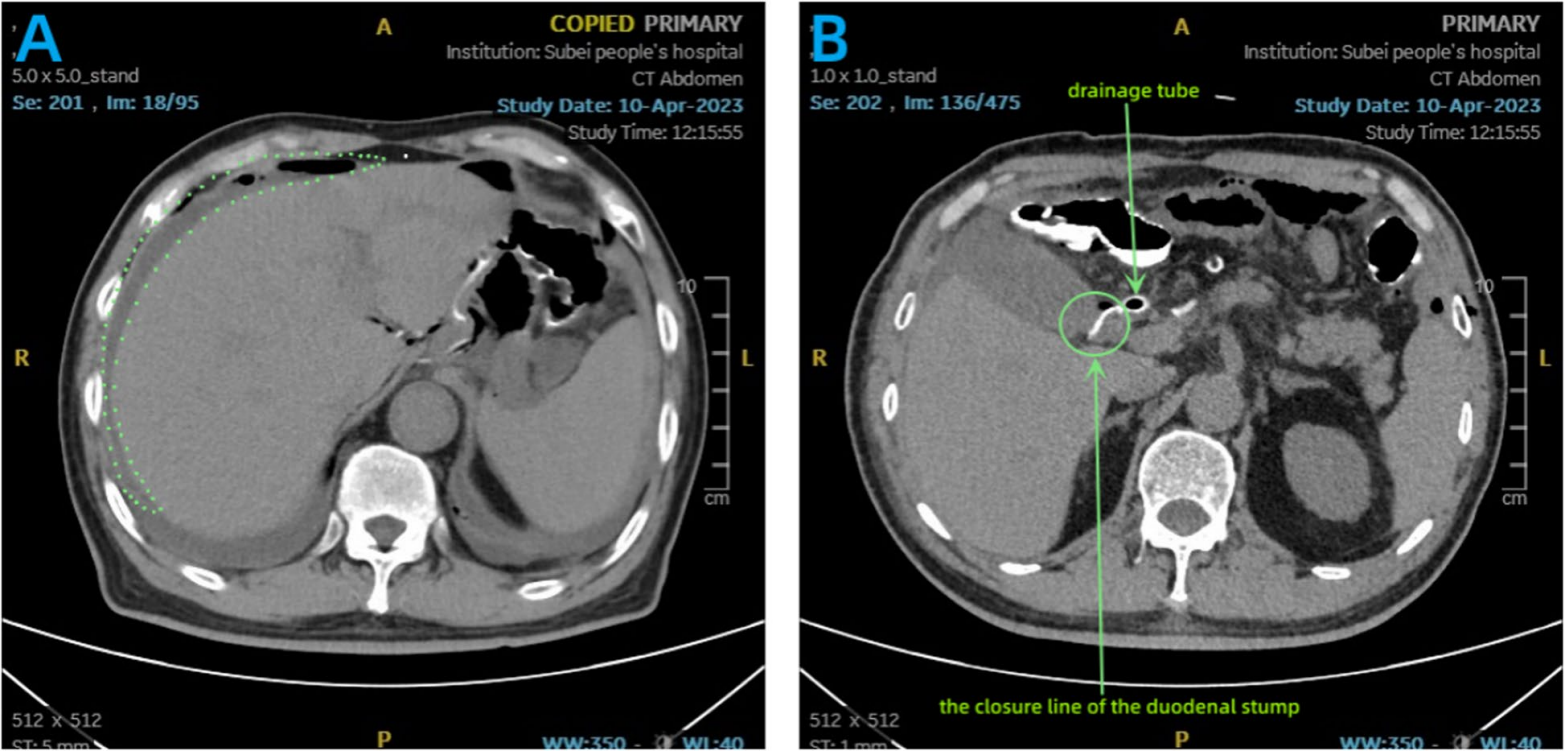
A Abdominal CT Scan of a Patient with Duodenal Stump Fistula: The dashed lines indicate the location where leaked fluids and gas accumulate around the liver. B Closure Line of Duodenal Stump in this DSL Patient: The closure line of the duodenal stump is depicted in the figure. It appears as a complete and intact line without any apparent defects
